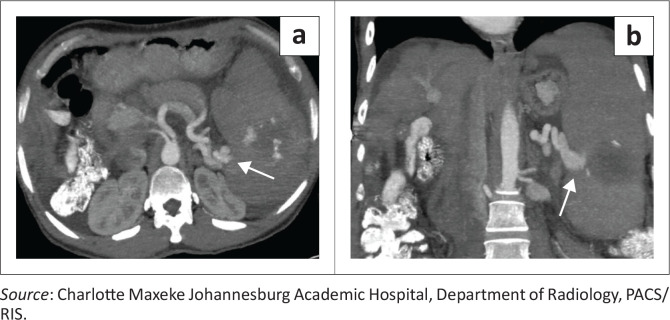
Splenic venous varix in a 44-year-old woman with portal hypertension who developed an enlarging splenic venous pseudoaneurysm that subsequently ruptured. (a, b) Axial and coronal contrast-enhanced CT images demonstrate a dilated tortuous splenic venous varix (arrows) with an associated intrasplenic haematoma. The splenic vein demonstrates early opacification during the arterial phase and the adjacent splenic artery is seen in close proximity, raising suspicion for an underlying vascular malformation.
